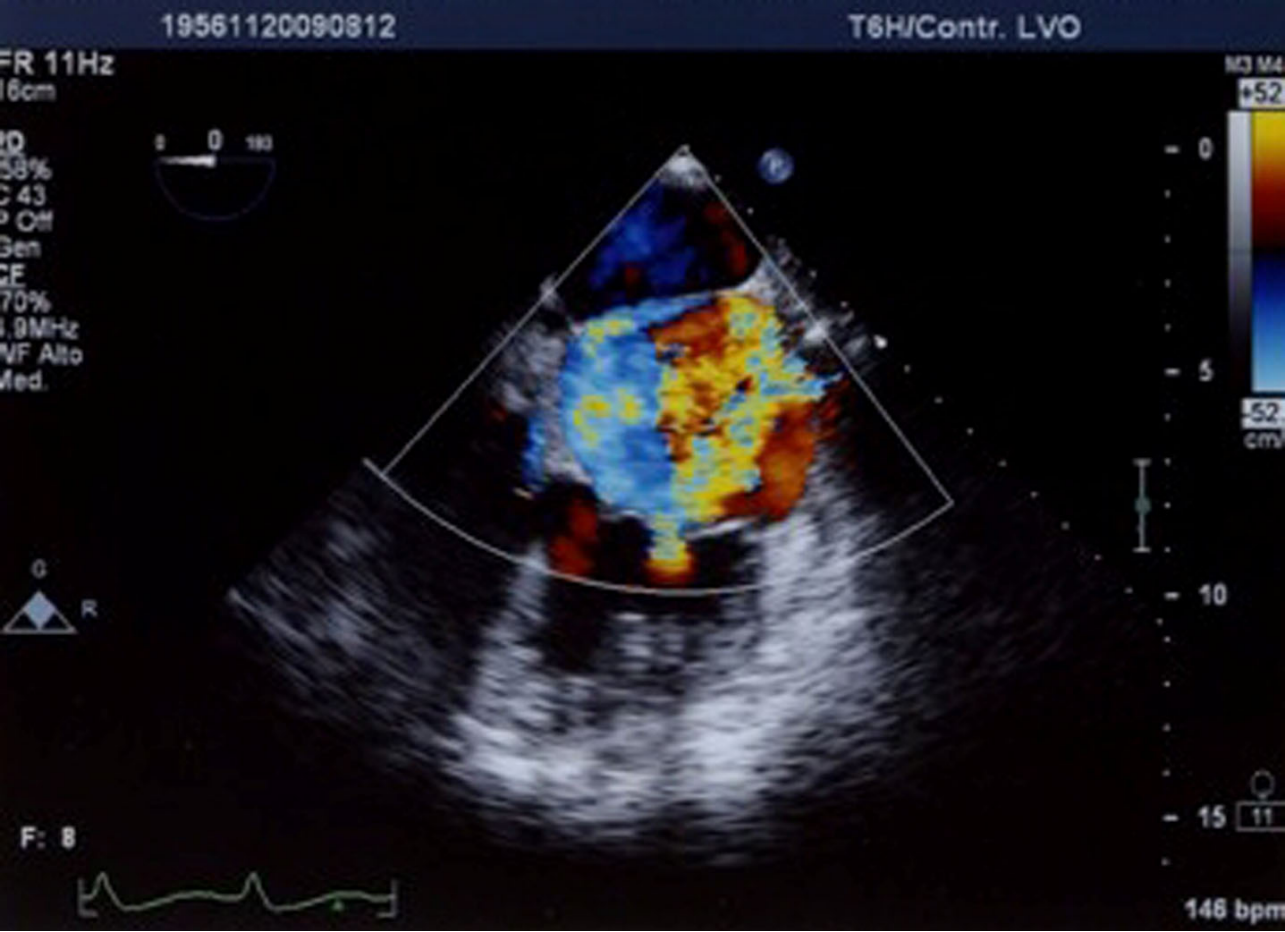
Transoesophageal echocardiogram: turbulent colour flow across the diaphragm in the left atrium, suggestive of an insufficiency from a single central opening.
